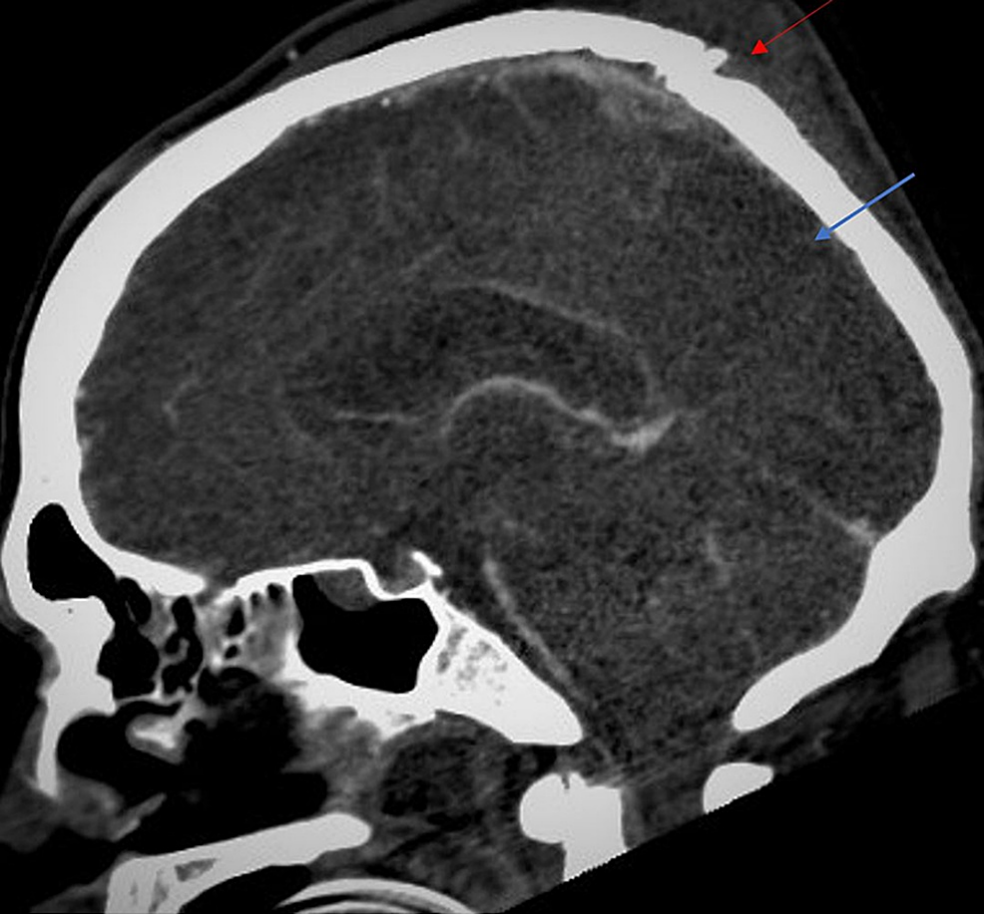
CT venogram on admission Red arrow: depressed skull fracture, blue arrow: non-opacification of contrast medium in posterior one-third of the superior sagittal sinus indicating an occlusive cerebral venous sinus thrombosis
